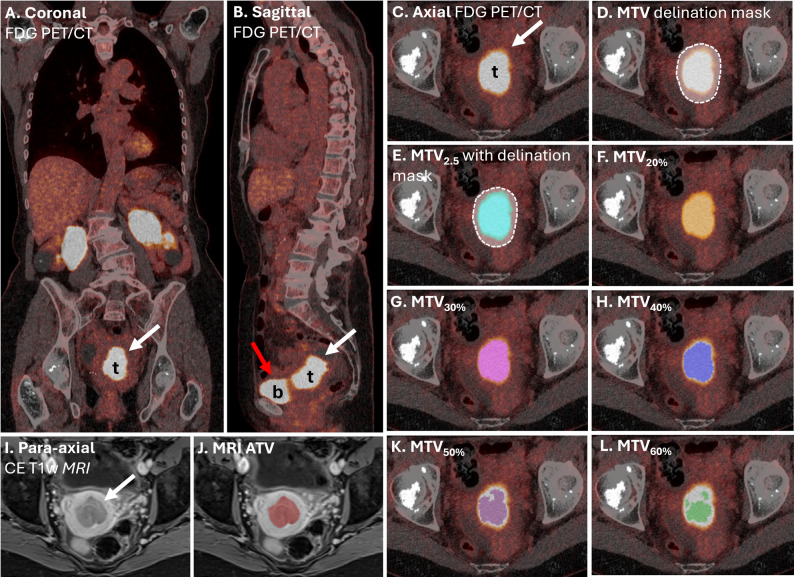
Metabolic and anatomical tumor volume segmentation. [^18^F]FDG PET/CT images in coronal (A), sagittal (B), and axial (C) views depict a primary endometrial tumor (t, white arrows) in a patient diagnosed with grade 2 endometrioid endometrial carcinoma, FIGO (2009) stage 3C1. The outer tumor mask (in gray with dotted line, D-E) was manually delineated slice-by-slice to encompass the tumor while excluding adjacent physiological uptake, such as from the bladder (b, red arrow). Within this mask, different standardized uptake value (SUV) thresholds were applied to define metabolic tumor volumes (MTVs): SUV > 2.5 (E), and relative thresholds of SUV > 20% (F), 30% (G), 40% (H), 50% (K), and 60% (L) of SUV_max_. Additionally, para-axial contrast enhanced (CE) T1-weighted MRI shows the primary tumor (white arrow, I), with the segmented anatomical tumor volume (ATV) highlighted in red (J). Abbreviations: [^18^F]FDG, fluorodeoxyglucose; FIGO, the International Federation of Gynecology and Obstetrics staging system; MRI, magnetic resonance imaging; PET/CT, positron emission tomography combined with computed tomography
